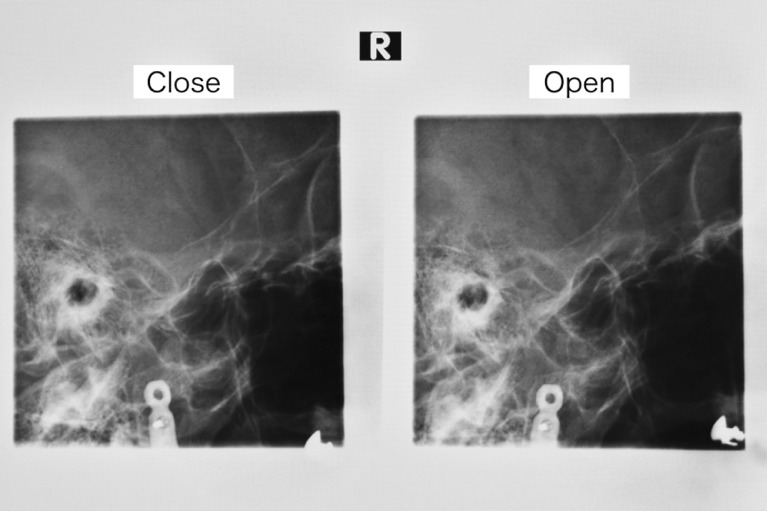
Patient 2—Temporomandibular joint radiograph. The distal end of the fibula is separated from the glenoid fossa.
